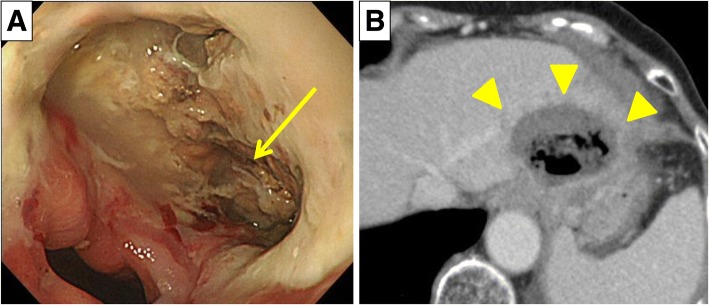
a Upper gastrointestinal endoscopy revealed perforation of the esophagus, and an ablated area of the liver was observed via the hole (arrow). b Contrast-enhanced computed tomography revealed that the cavity was localized due to severe adhesion (arrow heads)
